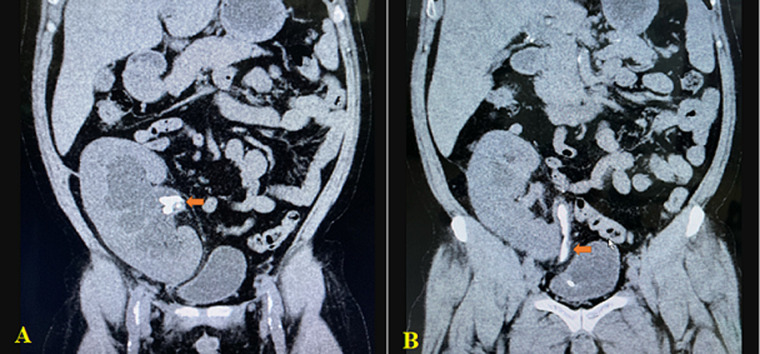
coronal computed tomography image showing stones in A) the pelvis of the transplanted kidney (arrow); B) the distal DJ stent (arrow)
